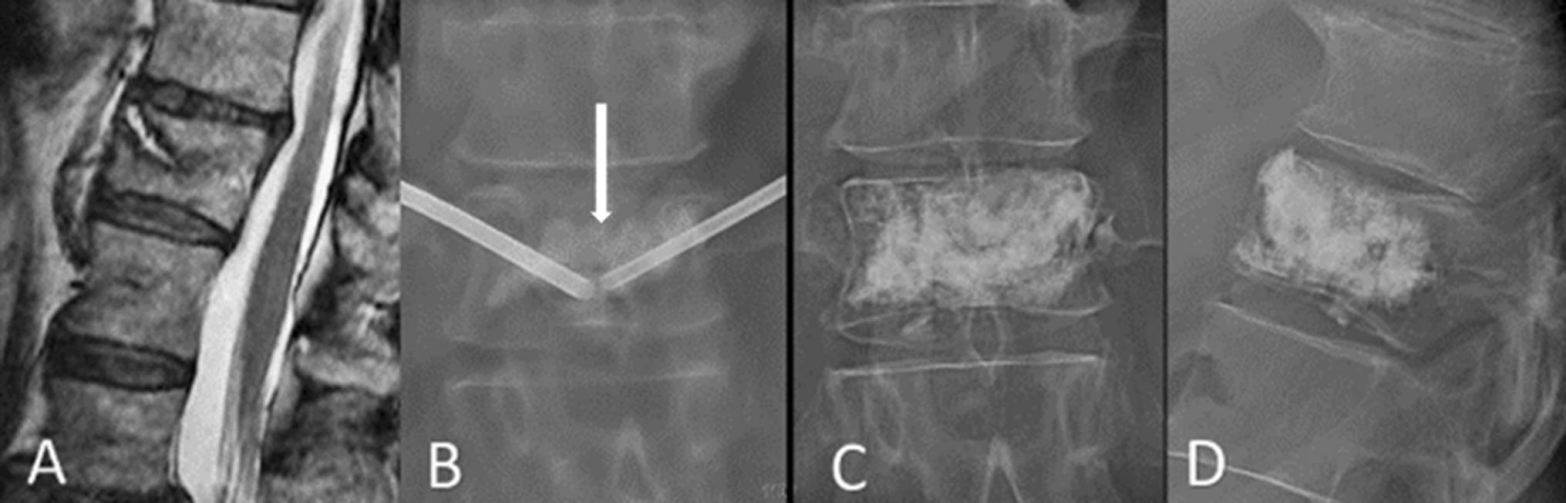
83-year-old female with 2 week history of severe back pain treated with vertebroplasty using “vertebral fill” technique. A Sagittal MRI shows oblique fracture cleft in T11. B Fluoroscopy image after injecting 3.5 cc of polymethyl methacrylate (PMMA) shows partial filling of the cleft (white arrow) but not the adjacent trabecular bone. C and D. AP and lateral images after vertebral fill injection of 8 cc PMMA. Cement is filling not just the fracture cleft but also supporting the trabecular bone above and below it to prevent ongoing fracturing. This is three times the mean PMMA volume injected in the earliest published blinded trials [1, 9], but similar to the mean volume used in the VAPOUR trial [3]
